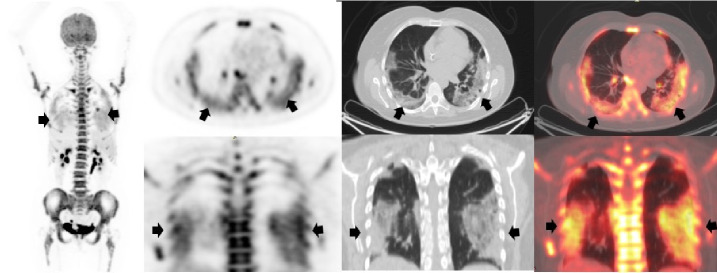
PET/CT F18-FDG en la cual se observó engrosamiento difuso con actividad metabólica irregular y SUVmáx de 3,7 en la pared gástrica de la topografía del antro y del cuerpo y el antro relacionada con el compromiso infiltrativo por la condición primaria conocida. En el segmento pulmonar (flechas negras) se evidenciaron múltiples opacidades con patrón de vidrio esmerilado, hipermetabólicas y dispersas en ambos parénquimas pulmonares, de localización periférica, SUVmáx de 6,5, de aspecto inflamatorio e infeccioso y sospechosas de COVID-19.
